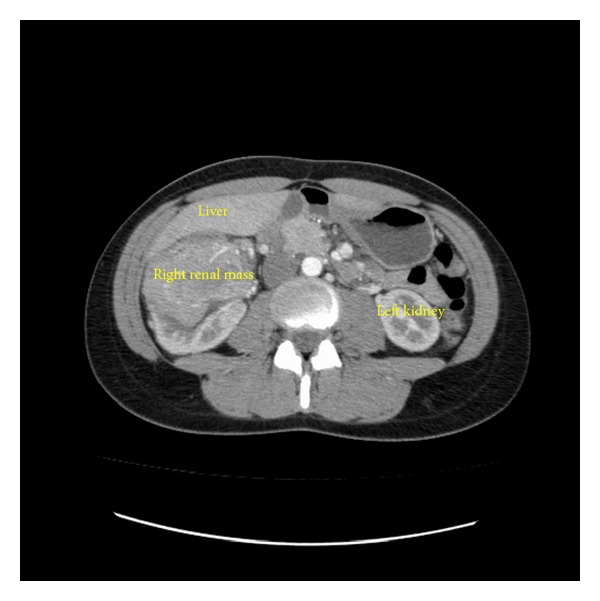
Axial images of computed tomography scan showing 9 cm right upper pole mass.
